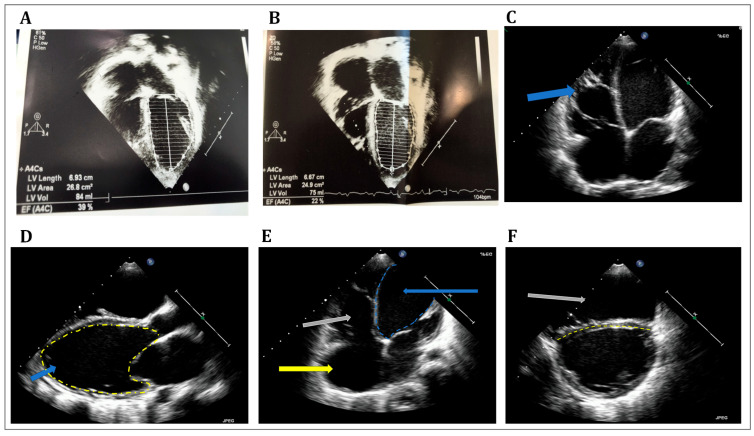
Echocardiography of affected siblings. (A) KU-48.IV-8 shows moderate left ventricular systolic dysfunction. (B) KU-48.IV-9 shows severe left ventricular systolic dysfunction. (C) KU-48.IV-8 shows dilated left ventricle in parasternal long axis view. (D) KU-48.IV-8 shows trabeculated right ventricle (blue arrow) in apical four-chamber view. (E) KU-48.IV-8 shows biventricular (blue and grey arrows) and right atrial dilatation (yellow). (F) KU-48.IV-8 shows right ventricular dilatation (grey arrow) with septal bowing.
